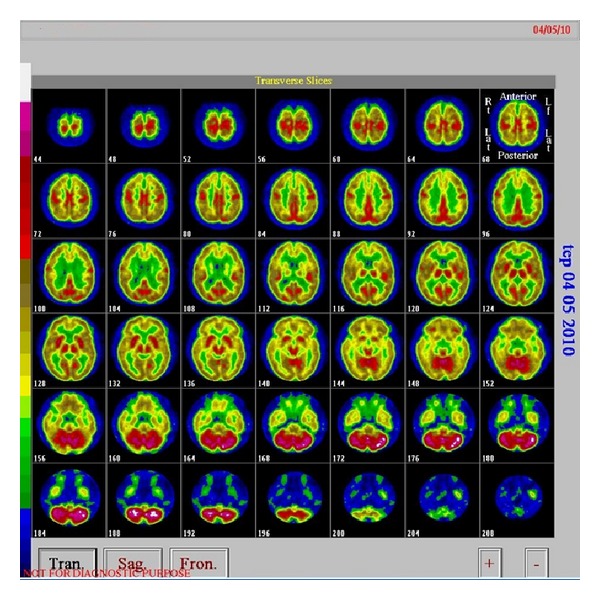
Brain 18-FDG-PET showing diffuse cerebral hypometabolism with cerebellar hypermetabolism (usually reported finding in PND).
